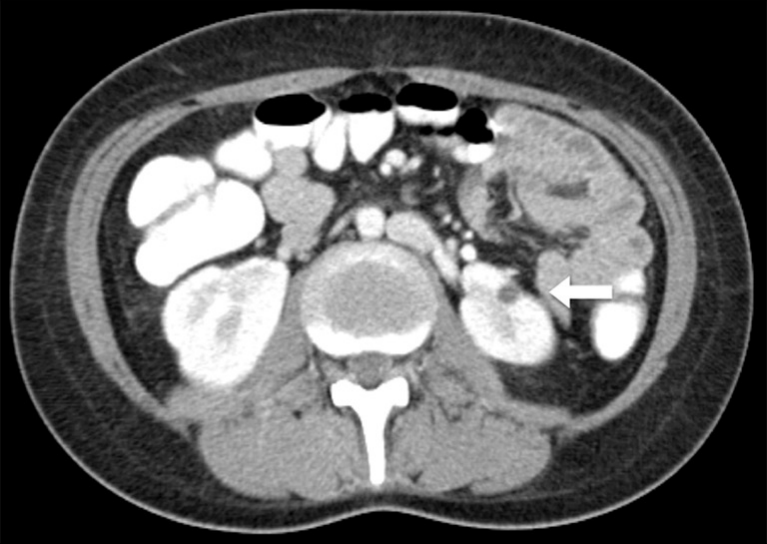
Axial CT scan in the portal venous phase shows a small focus of residual scarring in the left renal inferior pole (arrow) 6 months post initiation of prednisilone treatment.
